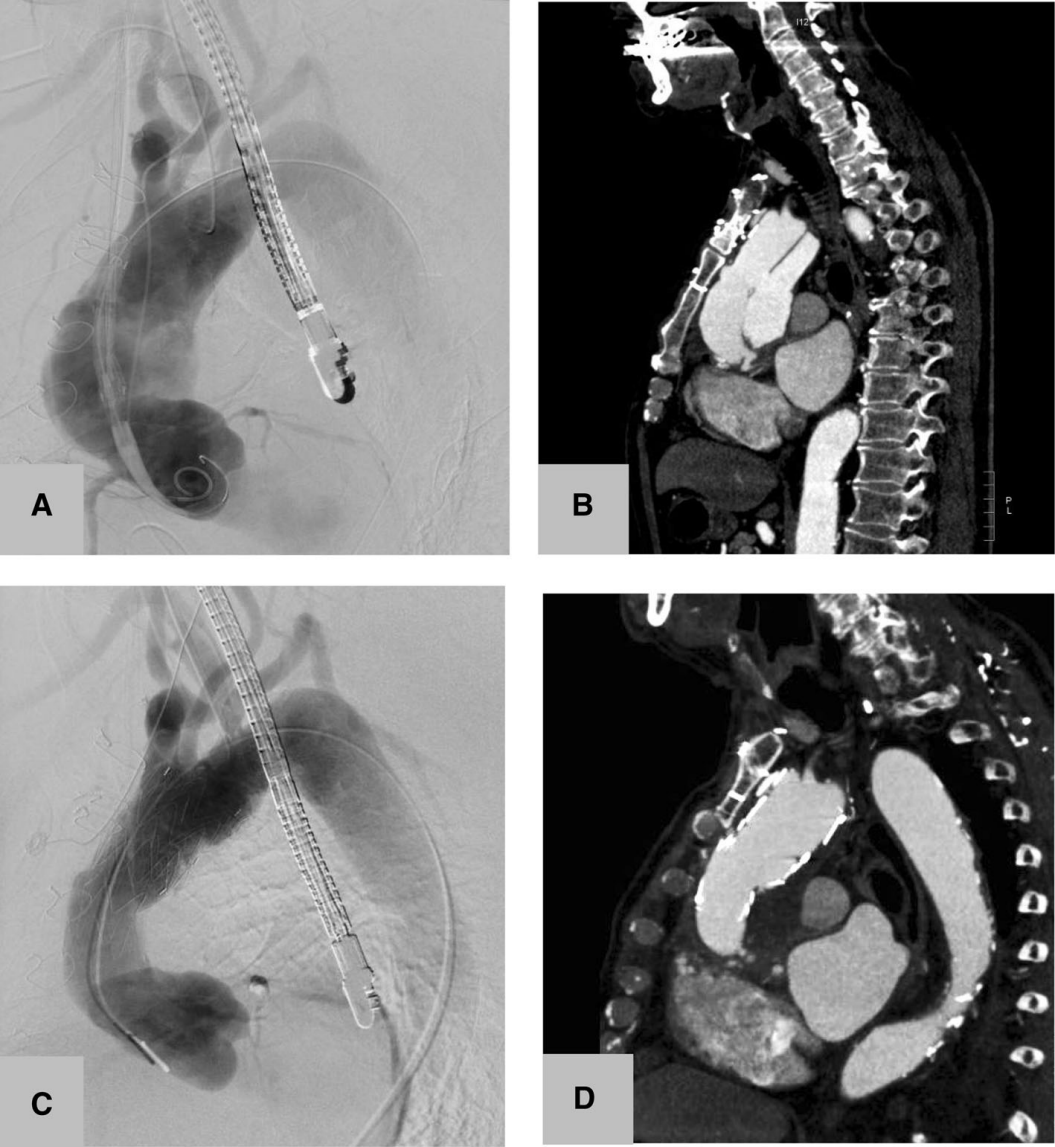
CT and digital subtraction angiography before (A, B) and after (C, D) exclusion of an ascending aortic pseudoaneurysm by deployment of 2 dedicated ascending aortic stent-grafts
